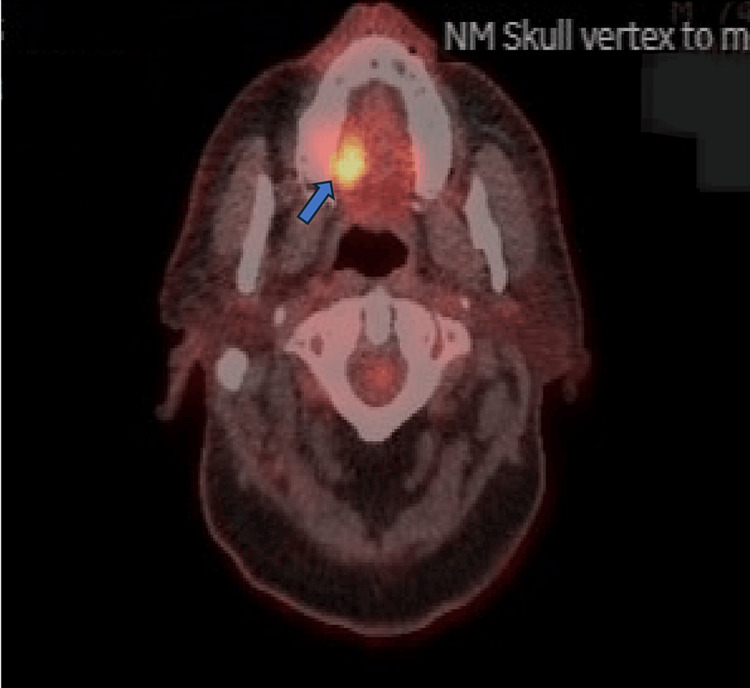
FDG avid lesion in the palate FDG: 18F-fluorodeoxyglucose
